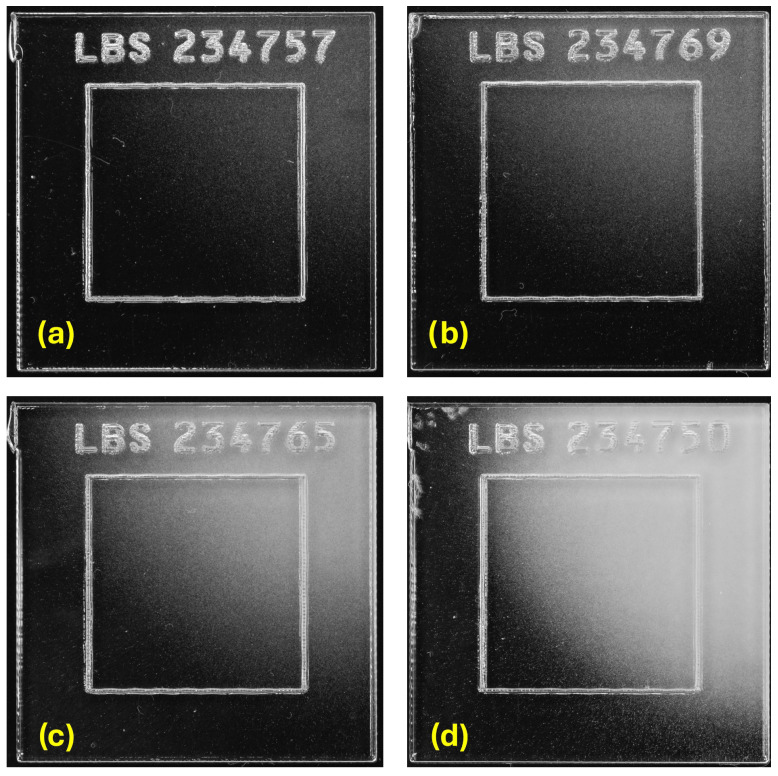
CR-39 dosimeters (after etching) irradiated at VESUVIO for 5 min (a), 10 min (b), 30 min (c), and 60 min (d).
